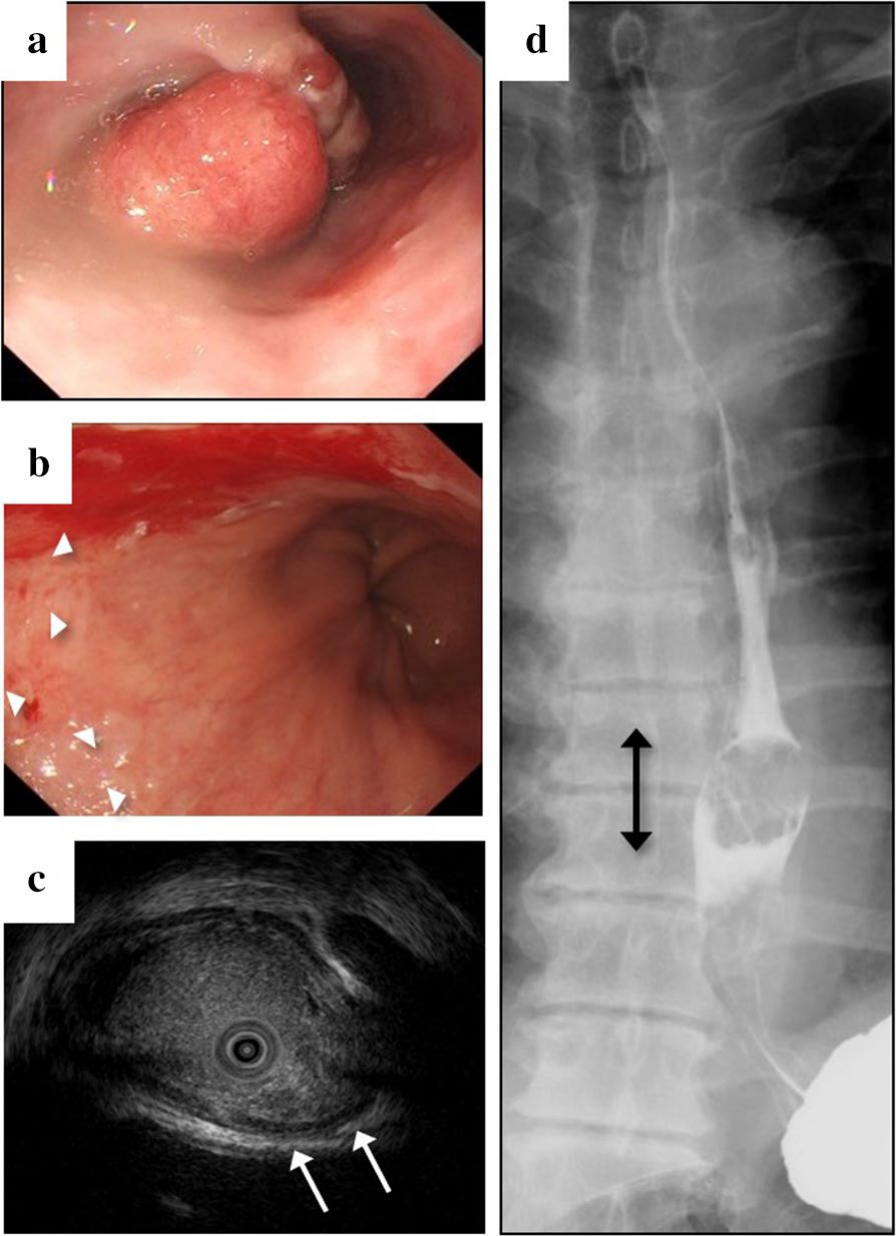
Upper gastrointestinal endoscopic and gastrointestinal contrast examination findings. A pendulous tumor approximately 2.0 cm in diameter was found at the left wall of the middle thoracic esophagus (a). Long segment Barrett’s esophagus extending about 4.0 cm in length was observed at the anal side of the tumor (arrowhead) (b). Endoscopic ultrasonography showed the tumor infiltrating into the deep submucosal layer (white arrow) (c). Gastrointestinal contrast examination showed a defect image of 2.2 cm in the lower thoracic esophagus (double-ended black arrow) (d)
